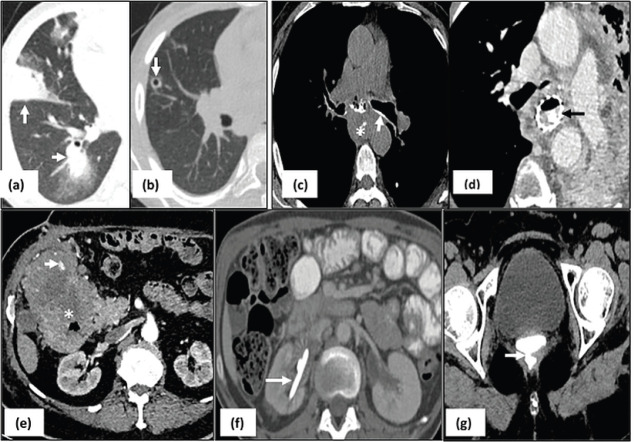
Interventions for management of CrC:leukemia patient with fungal pneumonia on HRCT image (arrows in a) responded to antifungal drugs evident as resolution of nodules and ground glass opacities with appearance of internal cavitation (arrow in b). Another patient having carcinoma esophagus with formation of trachea-esophageal fistula seen as oral contrast tracking in the airways (arrow in c) underwent esophageal stenting (arrow in d). Similarly, patient with intratumoural bleed (arrow) had to undergo embolisation for hemodynamic stability (e) and PCN (arrow in f) was done for urinary diversion in patient with hydronephrosis (same patient as in Figure 1b) before chemotherapy in order to improve the renal function status. On the other hand, post radiation rectovaginal fistula in a female with carcinoma cervix (arrow in g) was kept under close surveillance with conservative approach.
